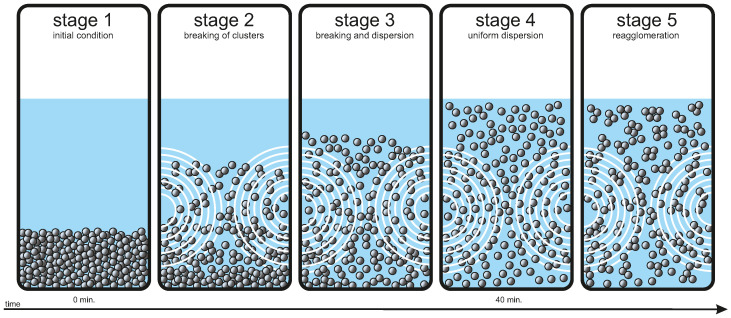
Various stages of nanofluid during ultrasonication.
